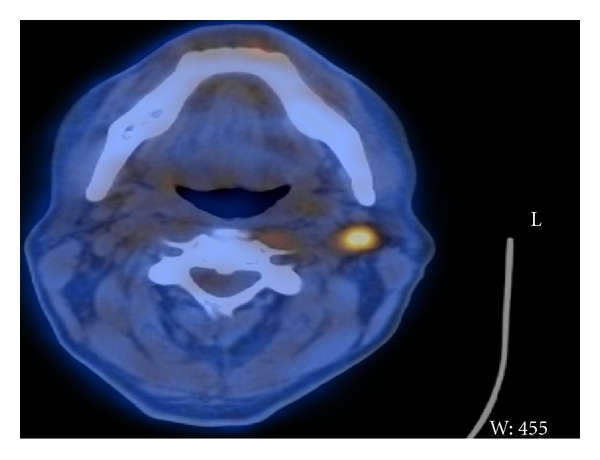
FDG-PET/CT scan performed three months after completion of chemoradiotherapy showed pathological uptakes in the left cervical lymph nodes.
